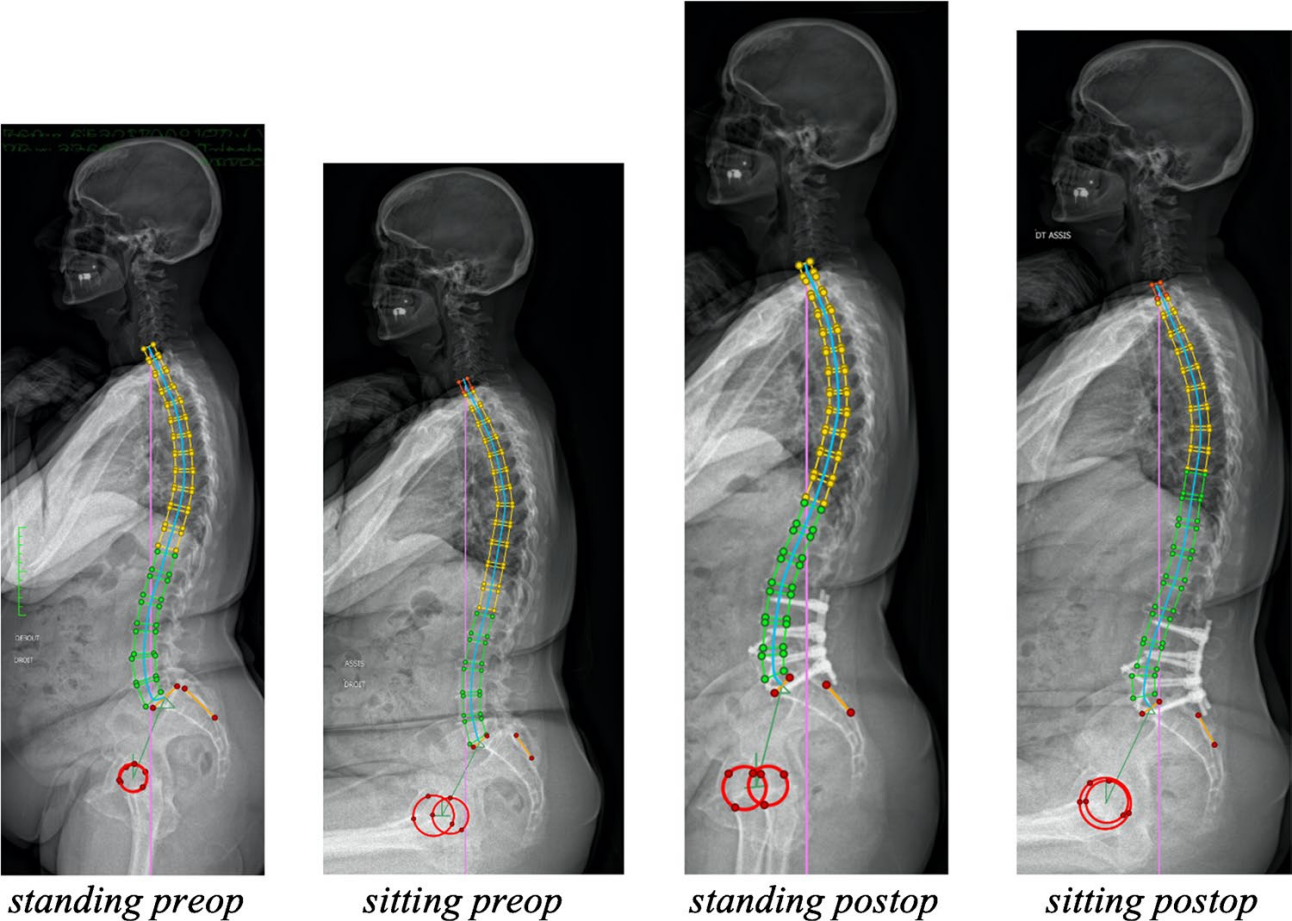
Radiographs by EOS and reconstruction with Keops® software
